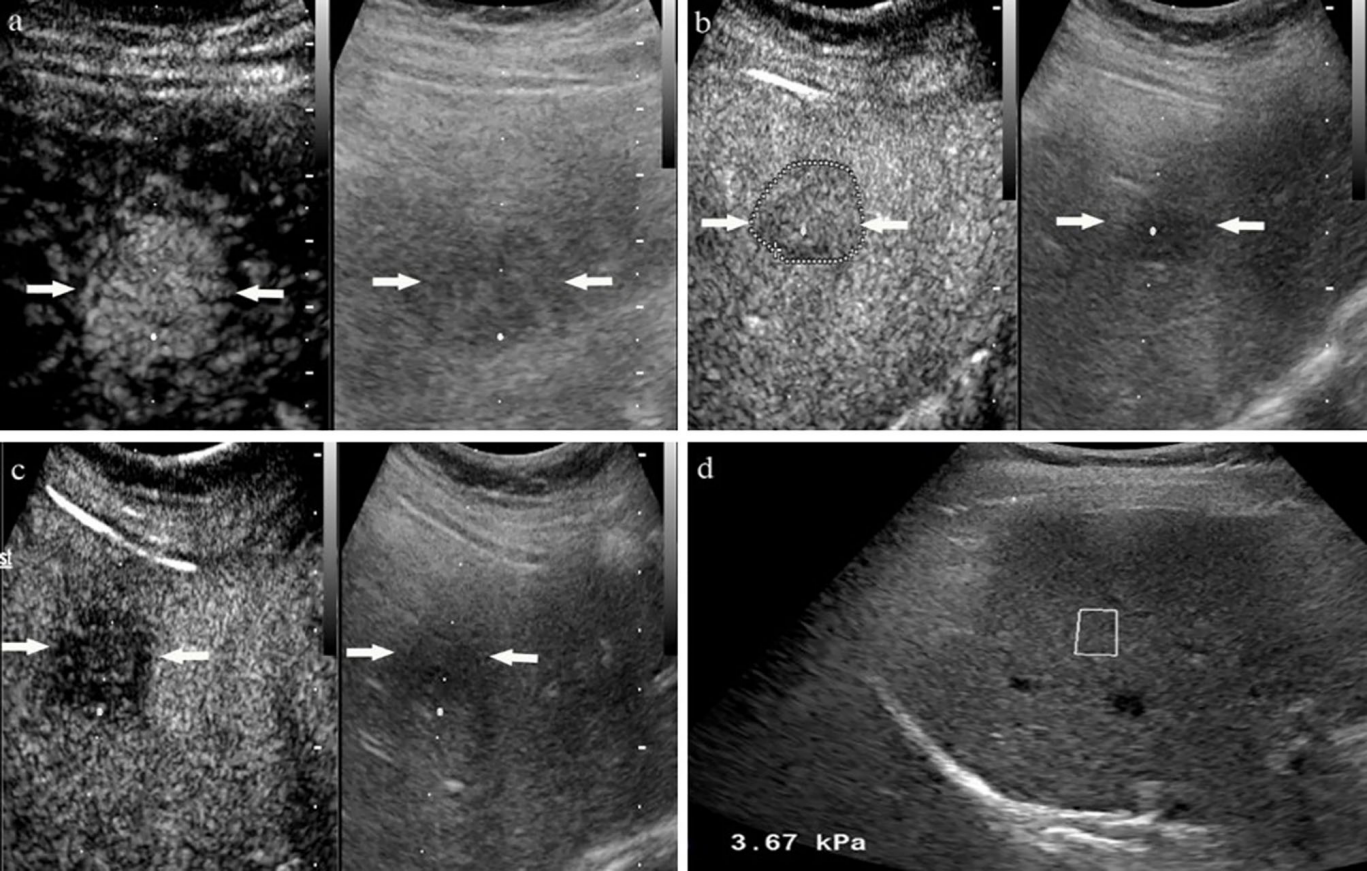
A 54-year-old woman with non-chronic hepatitis B virus complained of right epigastric discomfort. A hypoechoic tumor with largest diameter of 2.8 centimeters in anterior segment of the liver. At the arterial phase of contrast enhanced ultrasound, the mass showed rapidly whole hyperenhancement (A), and began washout after 60 seconds (B), finally with slightly washout at late phase (C). Liver stiffness measurement of liver background by ultrasound elastography showed the liver stiffness was 3.67 kPa (D). The lesion proved to be inflammatory pseudotumor by pathology.
